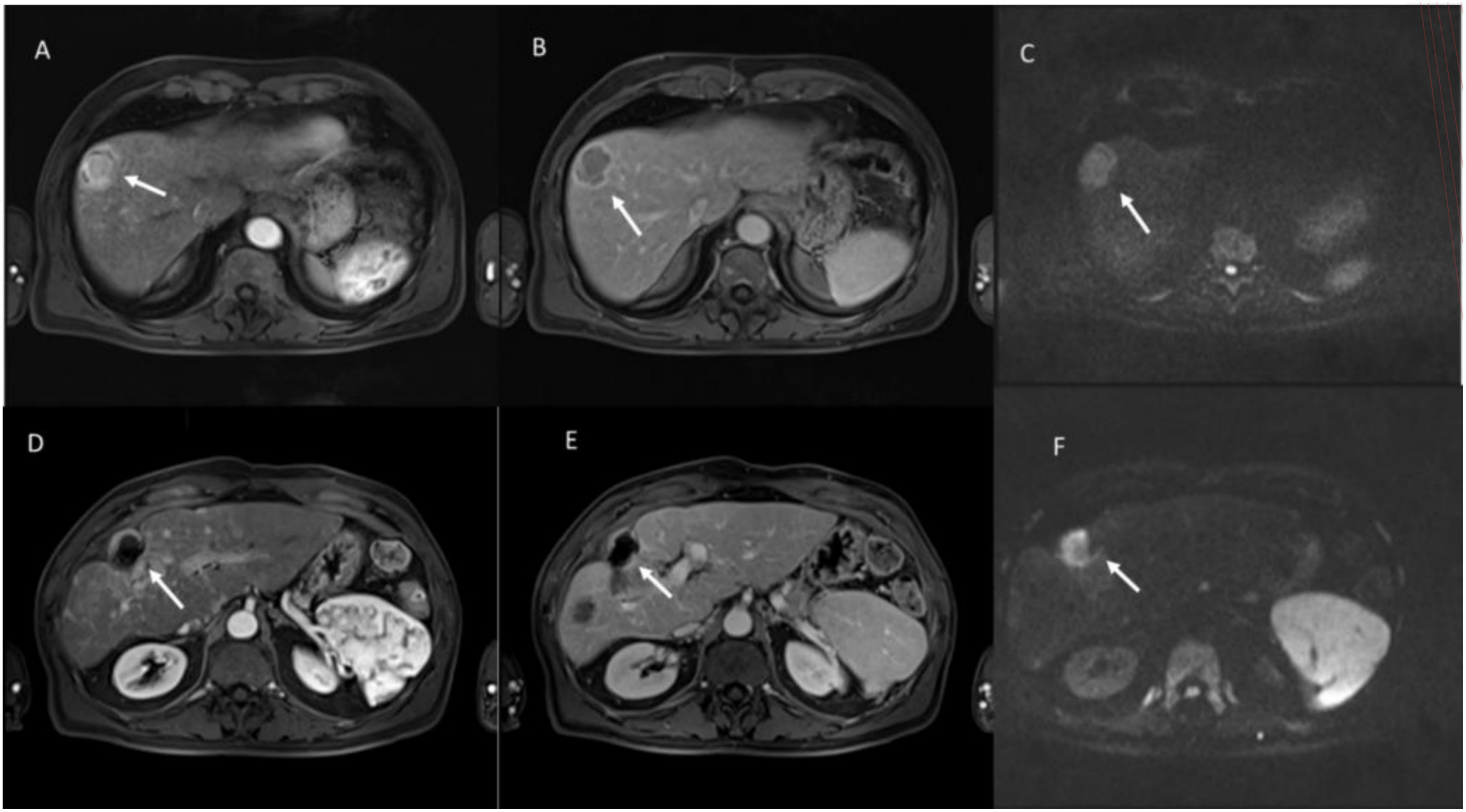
Multiparametric MRI assessment of evaluation of hepatocellular carcinoma (HCC) treated with radiofrequency ablation (RFA). Pre-Treatment Evaluation: (A) (Arterial Phase, T1-weighted VIBE FS): The lesion (white arrow) shows intense arterial hyperenhancement, a hallmark of viable HCC due to neovascularization. (B) (Portal Venous Phase, T1-weighted VIBE FS): The lesion exhibits washout, appearing hypointense compared to the surrounding liver parenchyma. (C) (Diffusion-Weighted Imaging, b800 s/mm²): The lesion demonstrates high signal intensity, indicating restricted diffusion due to high cellular density. Post-Treatment Evaluation (After RFA): (D) (Arterial Phase, T1-weighted VIBE FS): The lesion no longer exhibits arterial enhancement, with a hypointense center and peripheral rim, suggestive of post-ablation necrosis. (E) (Portal Venous Phase, T1-weighted VIBE FS): Persistent lack of contrast uptake confirms the presence of coagulative necrosis, indicating a successful ablative response. (F) (Diffusion-Weighted Imaging, b800 s/mm²): the treated lesion showed restricted diffusion for coagulative necrosis. From Vanzulli and Albano, (42).
